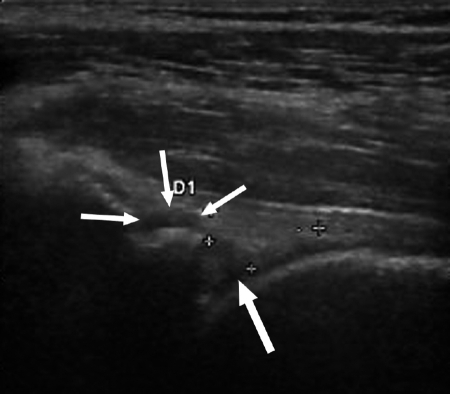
An acetabular labral tear visualized with ultrasound. The crosses mark the approximate limits of the triangular-shaped labrum. The thick arrow points to the hypoechoic cleft that runs through the base of the labrum, ending in cystic formation just superior to the labrum (3 thin arrows).
